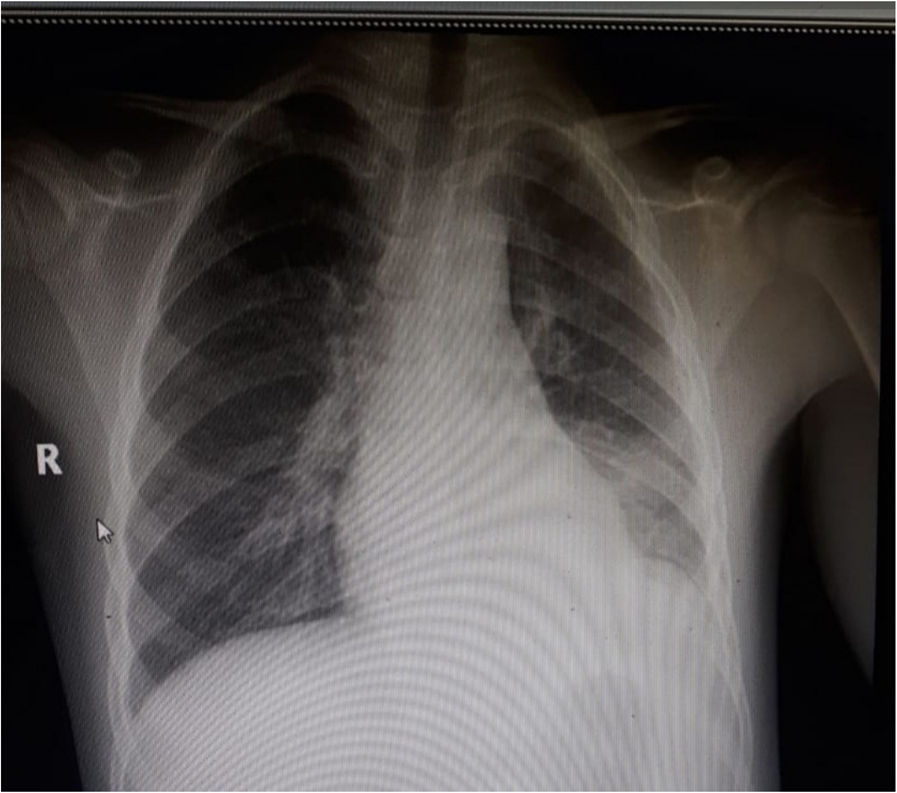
Chest X-ray of the patient showing left lower lobar consolidation with pleural effusion
